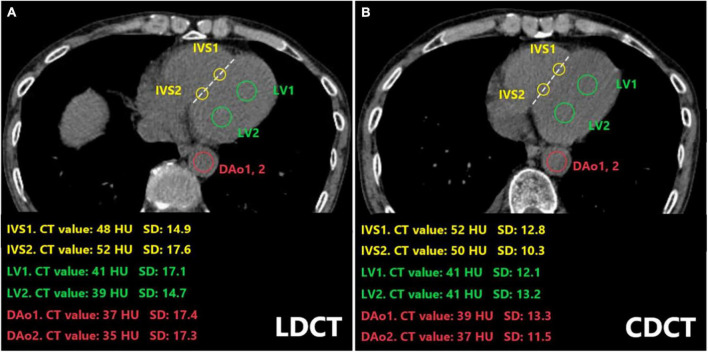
Placement of ROIs in the LV, IVS, and the DAo on CT images of a 64-year-old man who underwent both LDCT (A) and CDCT examination (B). The CT value and standard deviation for each ROI were indicated. ROIs on IVS were drawn along the line between the anterior interventricular sulcus and the anteriormost aspect of the left AV groove at the level of the junction of the coronary sinus and right atrium (dotted line). LDCT, low dose computed tomography; CDCT, conventional dose computed tomography; IVS, interventricular septum; LV, left ventricular cavity; DAo, descending aorta; HU, Hounsfield Unit; SD, standard deviation; ROI, Regions of interest.
